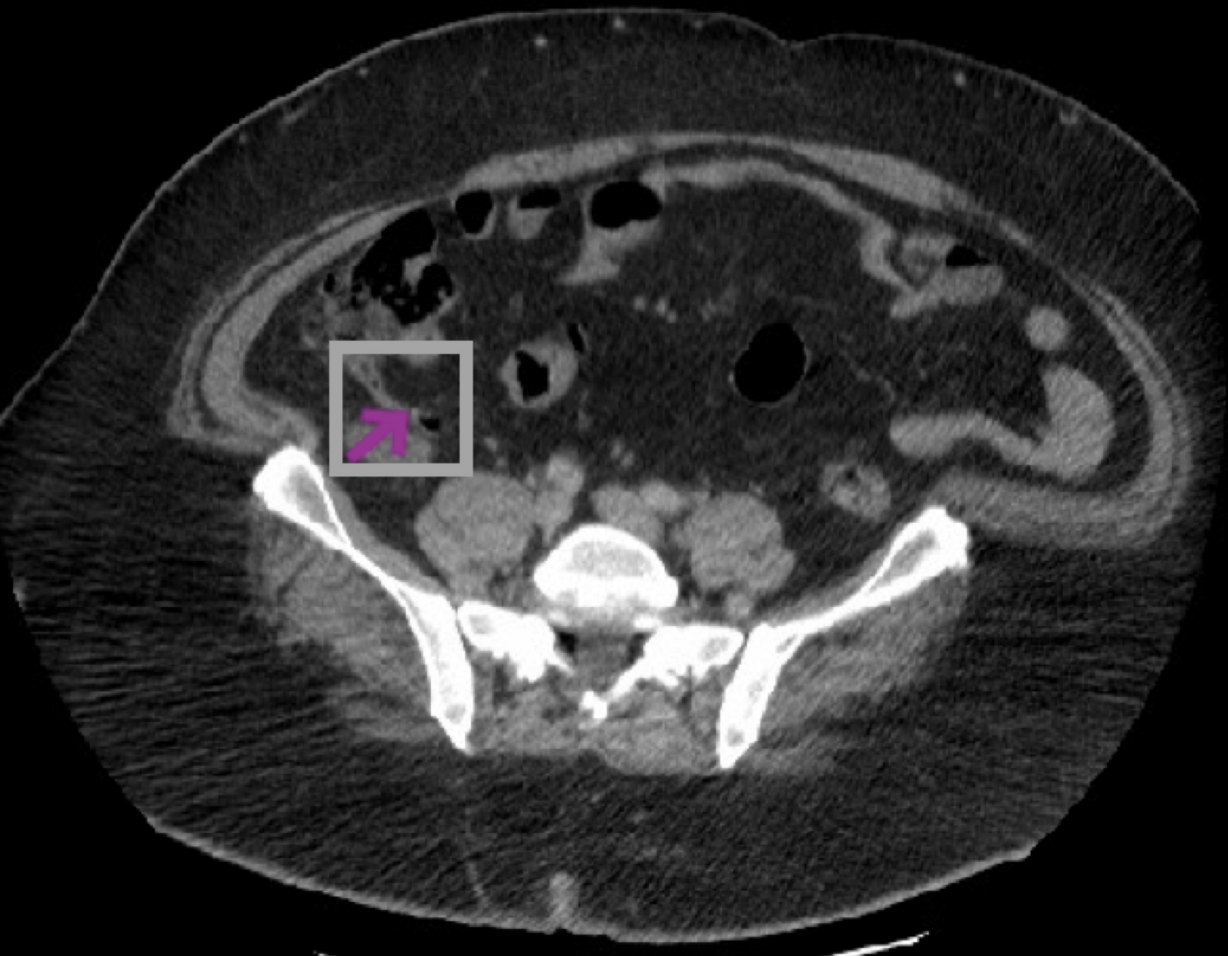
CT abdomen showed distended fluid-filled appendix CT: computed tomography
